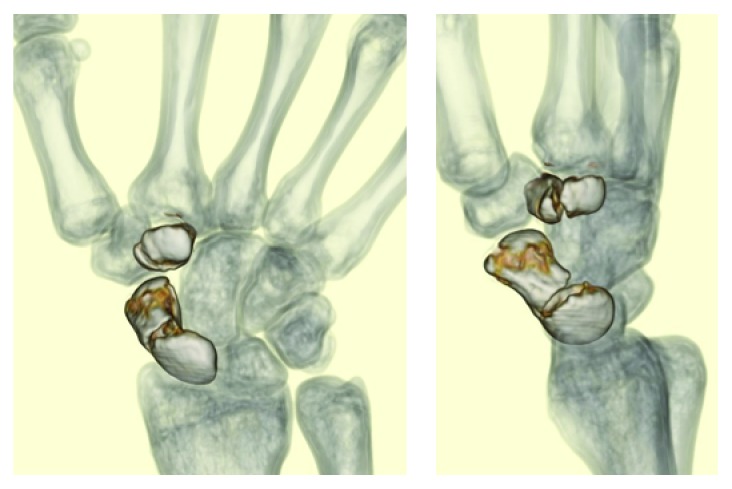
CT scan demonstrating fractures of the trapezium and the scaphoid.
